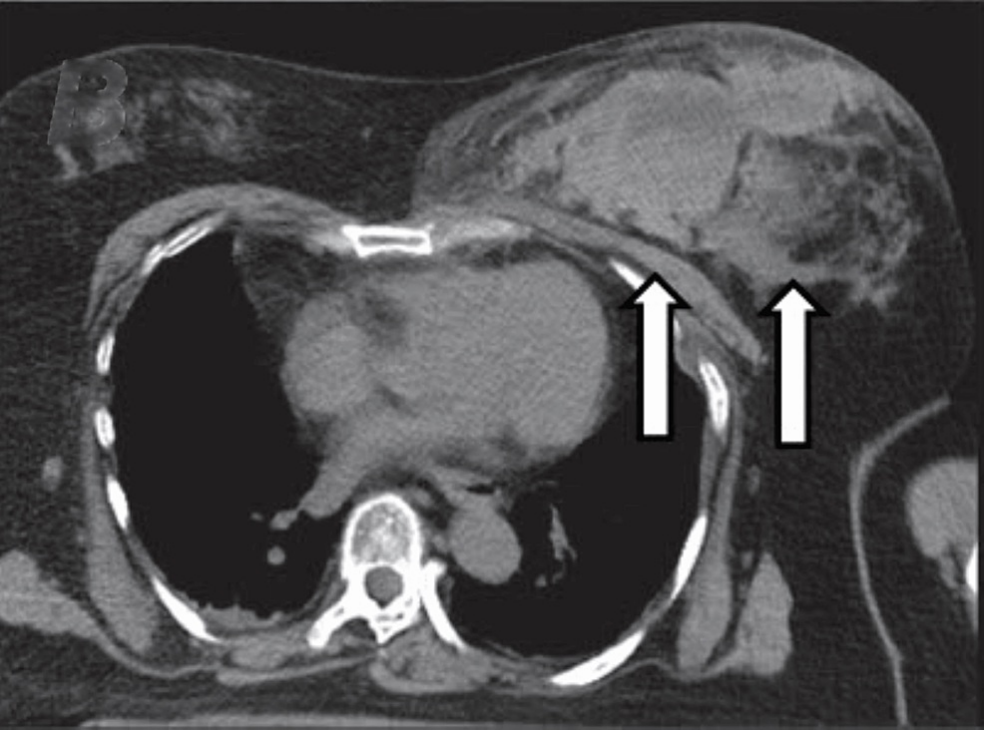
Plain computed tomography image. Plain computed tomography image of breast hematoma. A large breast hematoma is seen in the arrowhead area.
